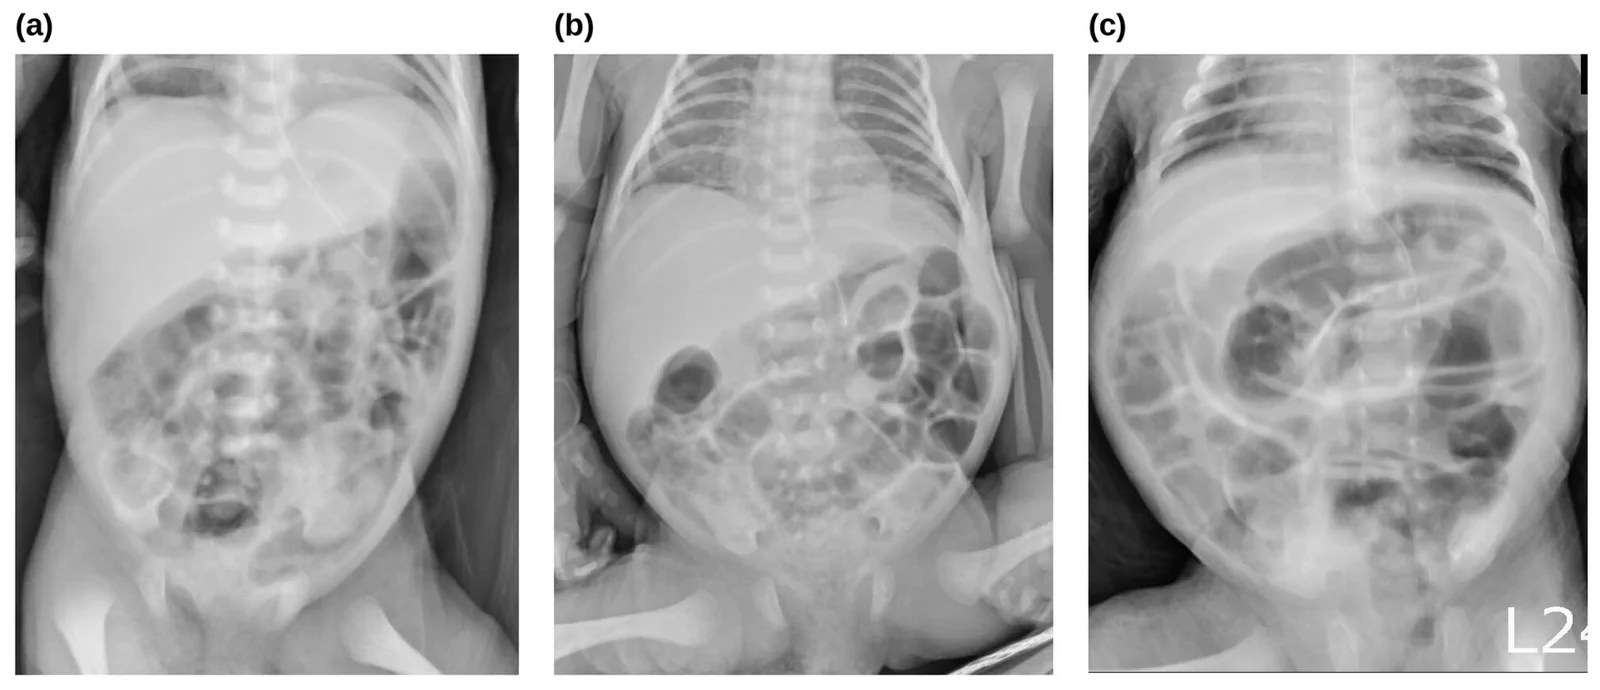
Serial anterior–posterior abdominal radiographic views demonstrating persistent, progressive generalized bowel dilatation since day 10. Views were performed at chronological age and PMA accordingly: (a) Day 10 (PMA 30 weeks), (b) Day 17 (PMA 31 weeks), and (c) Day 62 (PMA 37^+3^ weeks). PMA: Postmenstrual age.
